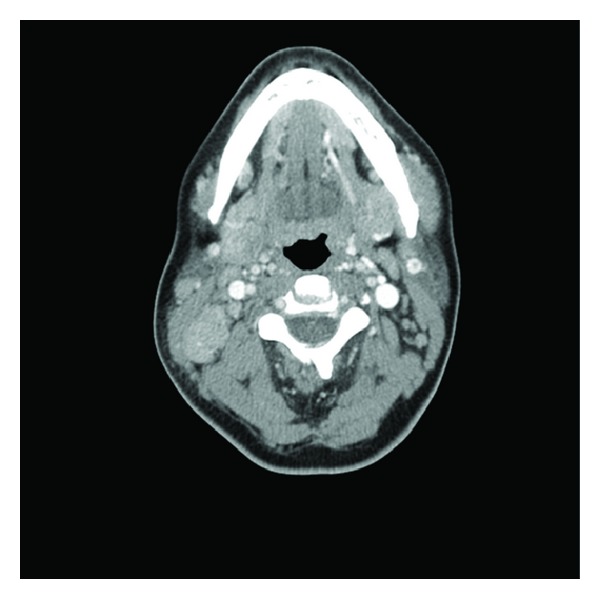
CT scan showing anterior and posterior cervical lymphadenopathy.
